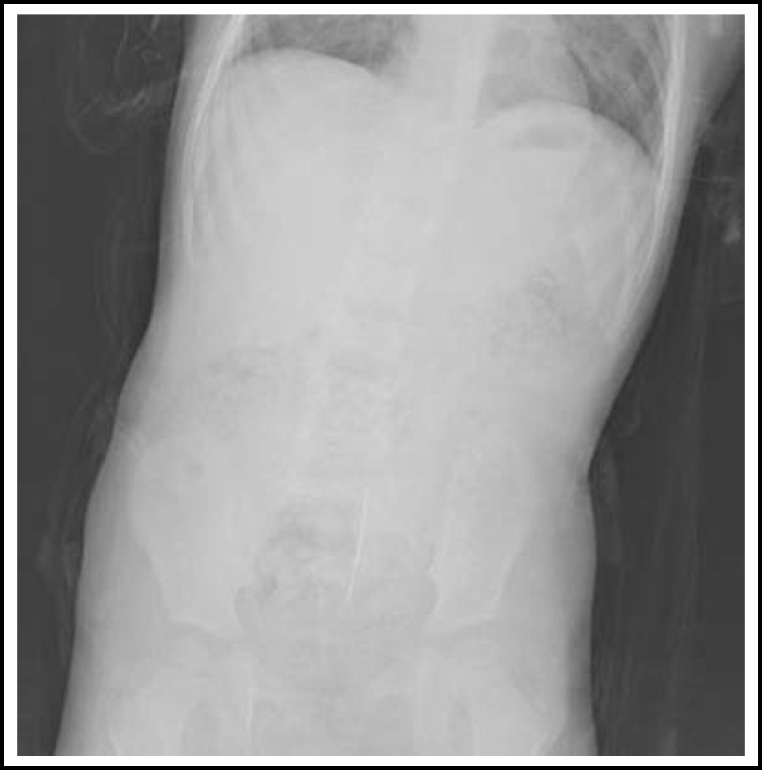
Abdominal radiograph shows a radiopaque foreign body in the lower abdomen
